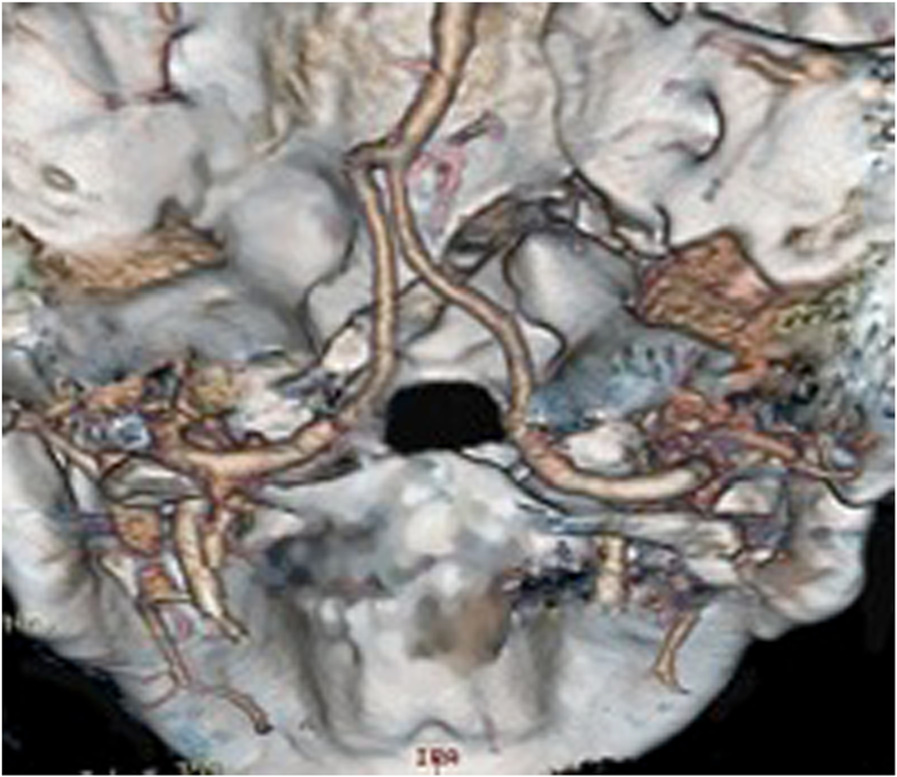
Computed tomography reconstruction of the craniocervical conjunction with fracture extending into the left occipital condyle.
